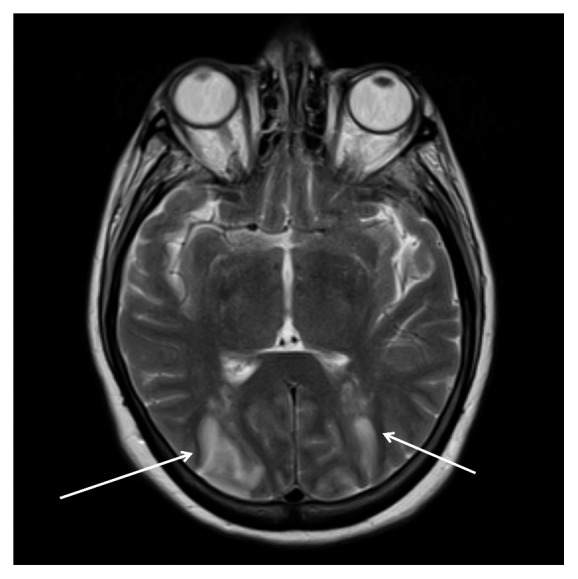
MRI T2-weighted sequence with hyperintense signal involving the occipital (arrows) and parietal lobes bilaterally, suggestive of posterior reversible encephalopathy syndrome.
